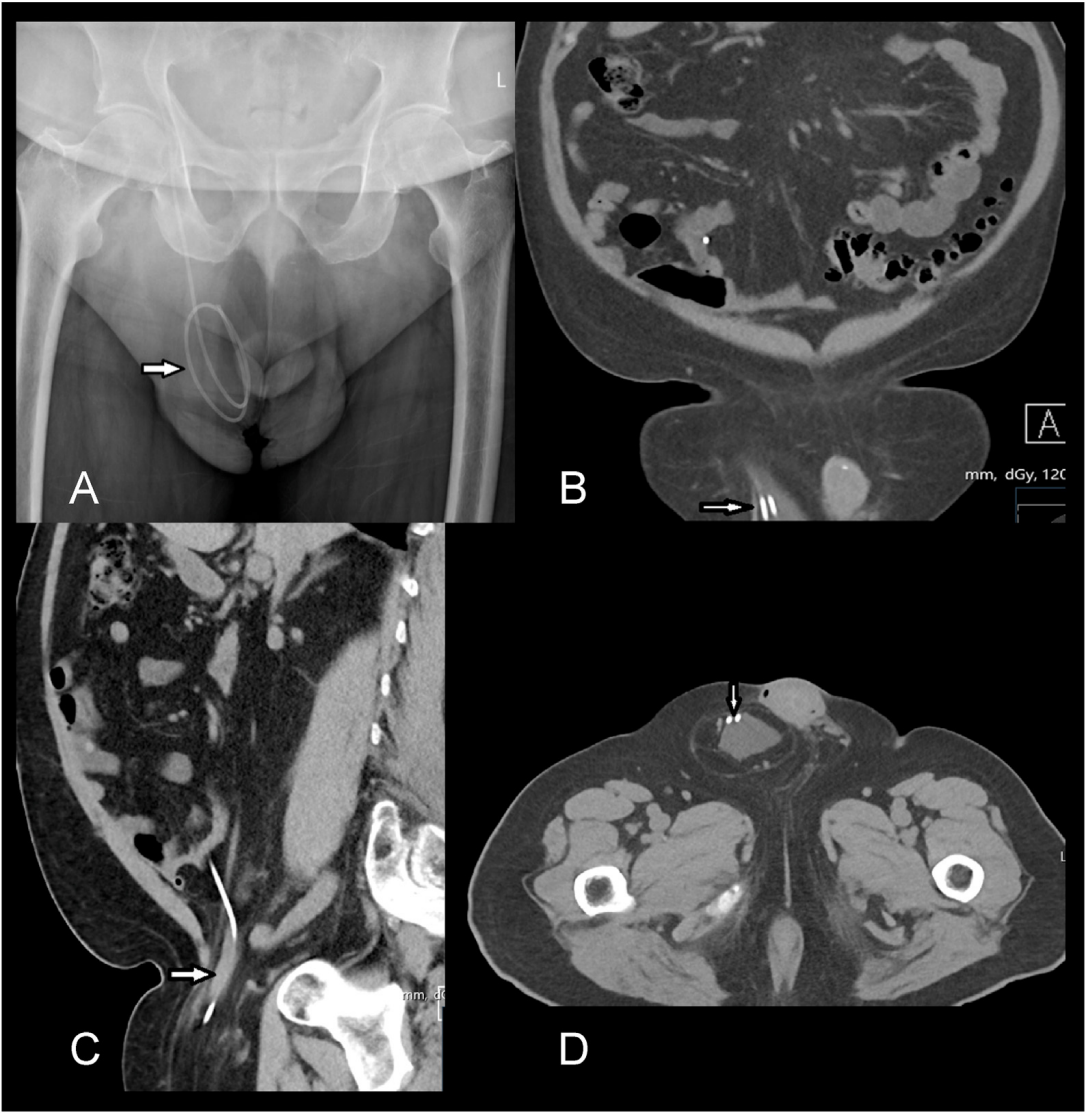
A, Preoperative AP abdominal radiograph showing the coiled distal shunt catheter inside the right scrotum (white arrow). B, coronal, C, sagittal and D, axial slices of abdominal CT scan showing distal shunt catheter in the hernia sac entering the scrotum.
